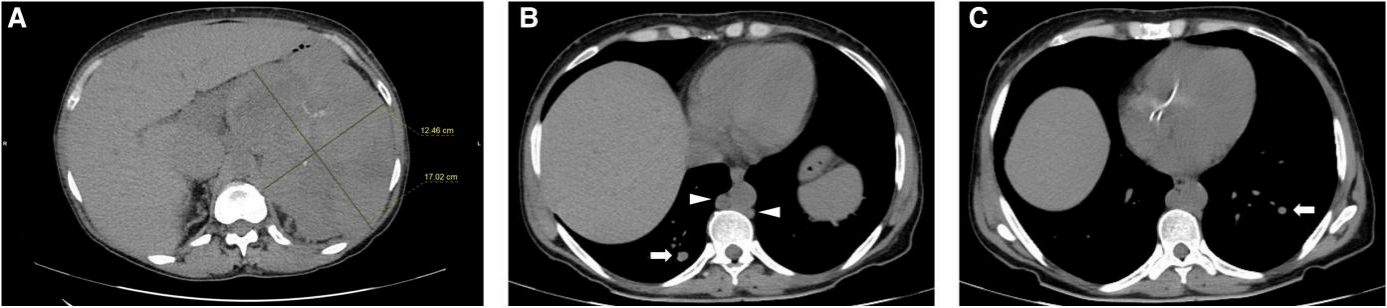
A non-contrast-enhanced abdominal computed tomography scan performed at the diagnosis shows a large solid mass of 17 cm with homogeneous and inhomogeneous areas located in the left adrenal lodge, encompassing the ipsilateral kidney (A), multiple bilateral lung nodules (white arrows), and para-aortic lymphadenopathy (white arrowheads) (B, C).
